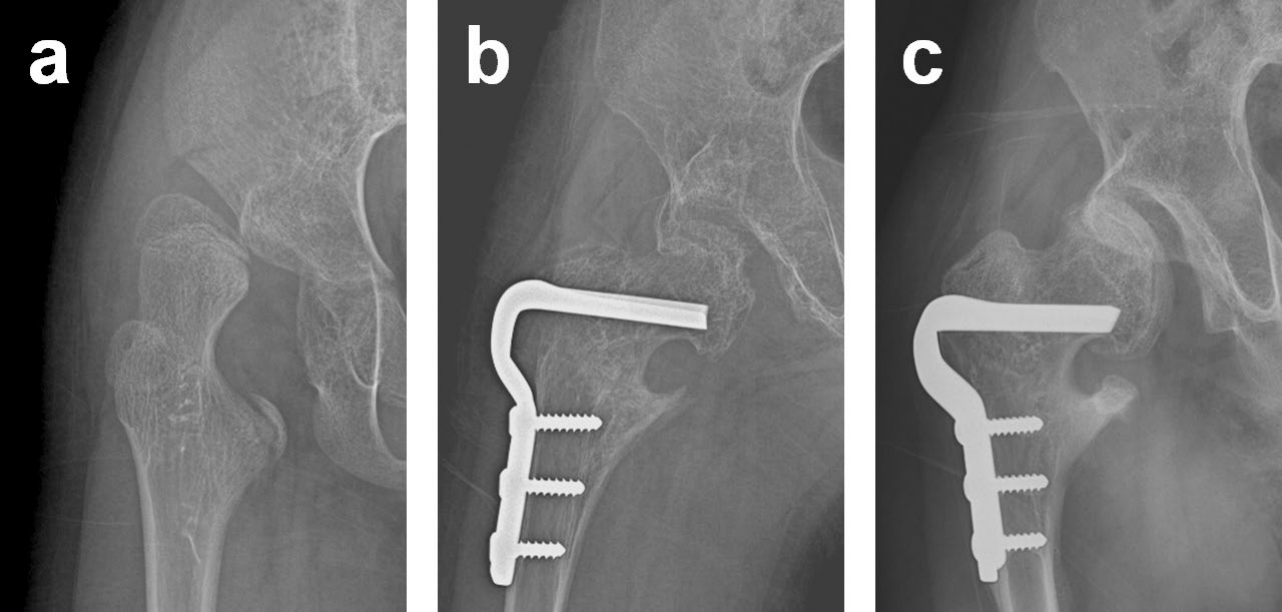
(a) Preoperative AP radiograph showing a dislocated hip in a 9-year-old girl. (b) AP radiograph showing Type 5 AVN developed after open reduction, VDRO, and pelvic osteotomy. (c) At 4 years after surgery, the femoral head failed to be remodelled and the hip was subluxated.
